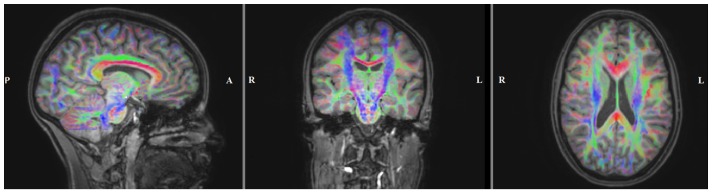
Diffusion directions obtained from DTI. Sagittal (left), coronal (middle) and axial (right) slice of the color coded fractional anisotropy (FA) map computed from the registered diffusion tensors and plotted on the registered T1w-MRI. The color indicates the main fiber orientation: red is left-right, green is anterior-posterior and blue is superior-inferior.
